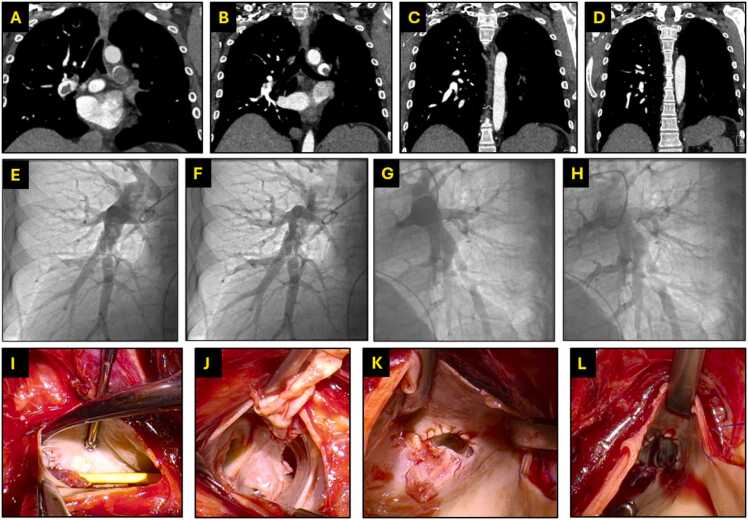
Preoperative CT-scans and intraoperative situs. A: acute thrombotic material in the right inferior lobe artery and in the left pulmonary artery with complete occlusion at the time point of acute PE. B: Follow-up imaging after 18 months shows organized thrombotic material in the right inferior lobe artery and in the left pulmonary artery. C & D: Preoperative CT-scan showing peripheral webs and bands on the right side and almost completely obliterated arteries on the left side. E & F: Preoperative pulmonary angiography of the right side. G & H: Preoperative pulmonary angiography of the left side. I & J: Chronic thromboembolic material in the right pulmonary artery during PEA. K & L: Thrombotic material in the left pulmonary artery appearing as a blind bag at the level of the former anastomosis. D: Desobliterated left pulmonary artery with unimpaired former anastomosis.
